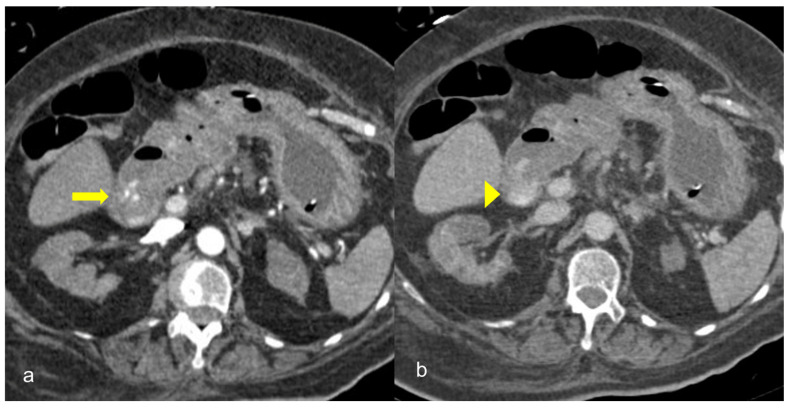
A 75-year-old intubated patient with COVID-19 presented with hematemesis, hypotension and acute drop of hemoglobin level. Computed Tomography revealed active intraluminal extravasation of contrast into the 2nd part of duodenum (arrow) on arterial phase (a). Further pooling of the contrast (arrowhead) is shown on portal phase (b).
